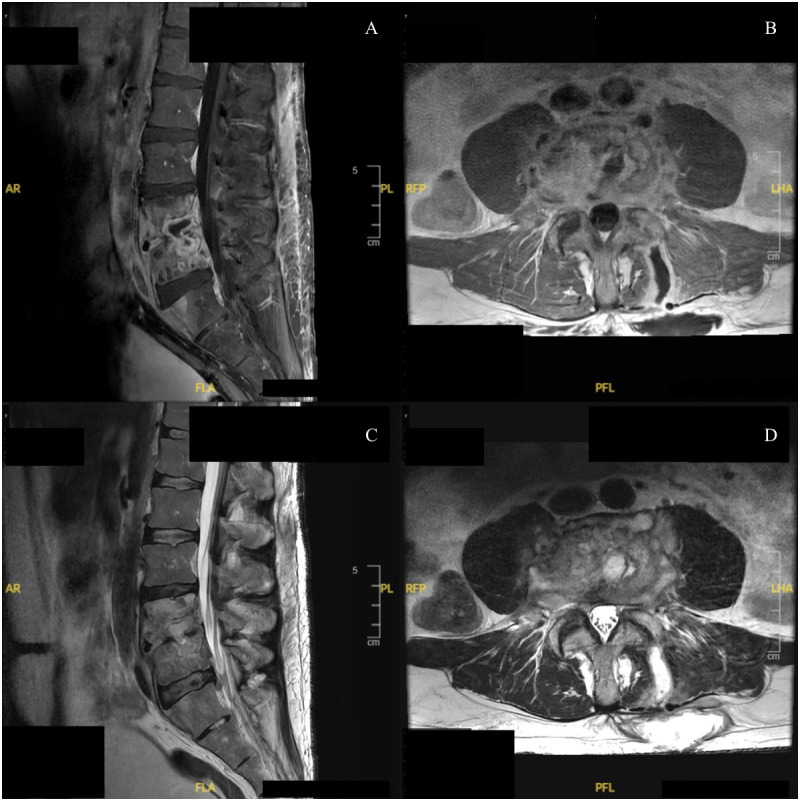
Day 29 MRI showing interval worsening. Repeat MRI on Day 29 after re-presentation with purulent drainage demonstrated persistent L4–L5 infection with progression of paraspinal, epidural, and foraminal abscesses. The left posterior L4–L5 fluid collection increased to 2.8 × 0.8 × 3.8 cm, with a new subcutaneous fluid collection extending from L1–L2 to L5–S1 measuring 3.8 × 1.6 × 13 cm. (A) Sagittal T1 post-contrast MRI, (B) axial T1 post-contrast MRI, (C) sagittal T2 image, and (D) axial T2 image. Alt text: Follow-up lumbar spine MRI (Day 29) showing progression of infection with persistent L4–L5 spondylodiscitis, enlarging paraspinal abscess, and new extensive subcutaneous fluid collection extending from L1–L2 to L5–S1, with involvement of epidural and foraminal regions (sagittal and axial T1 post-contrast and T2 images).
